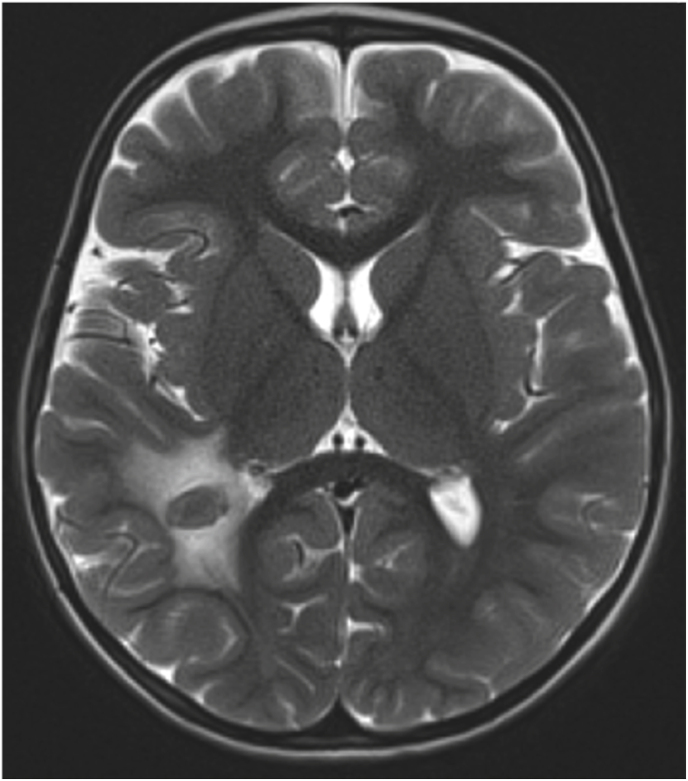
Post-contrast axial MRI showing a peripherally enhancing lesion in the right temporoparietal region with restricted diffusion and prominent vasogenic edema.
